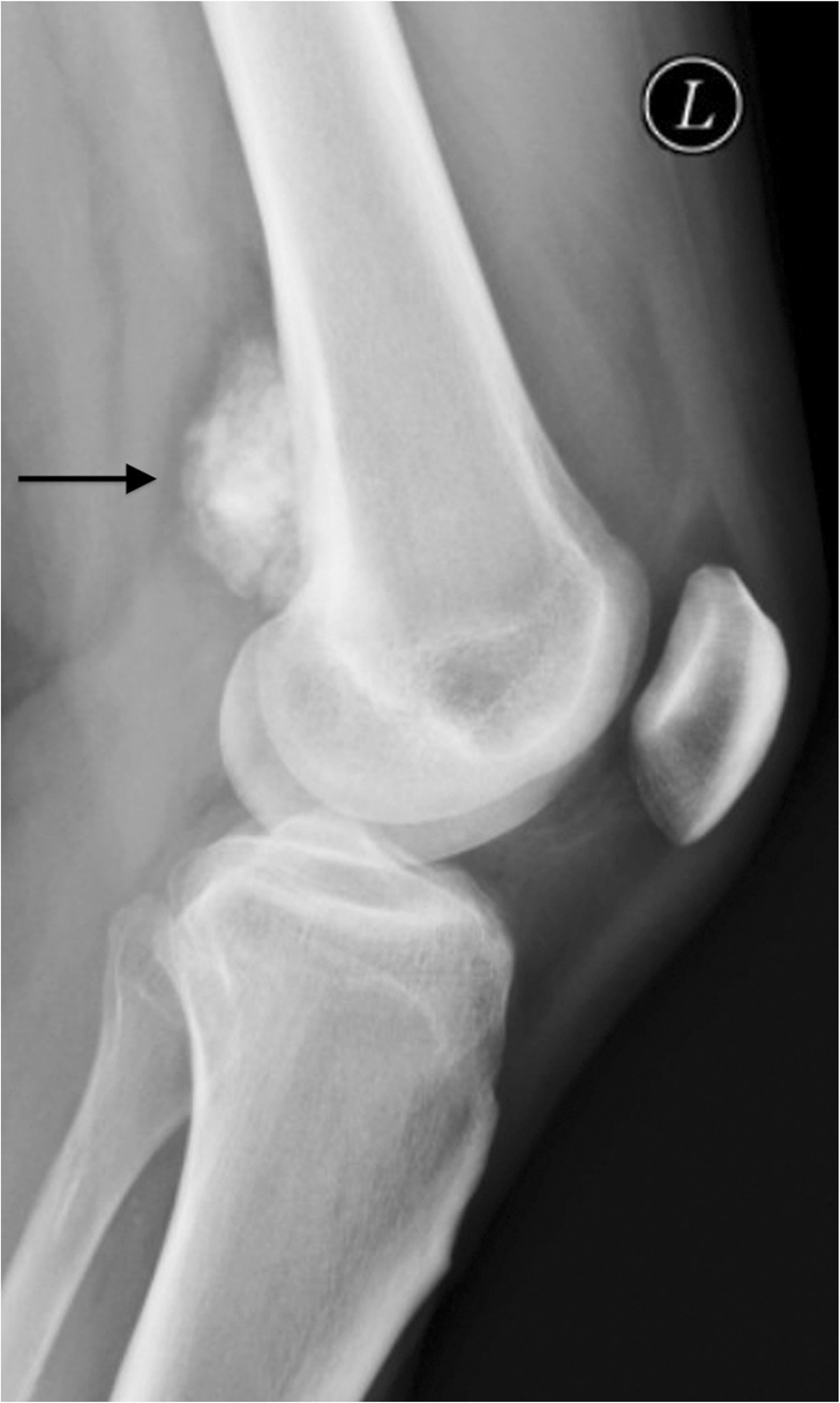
Exemplary lateral radiograph of a parosteal osteosarcoma, loco typico.
